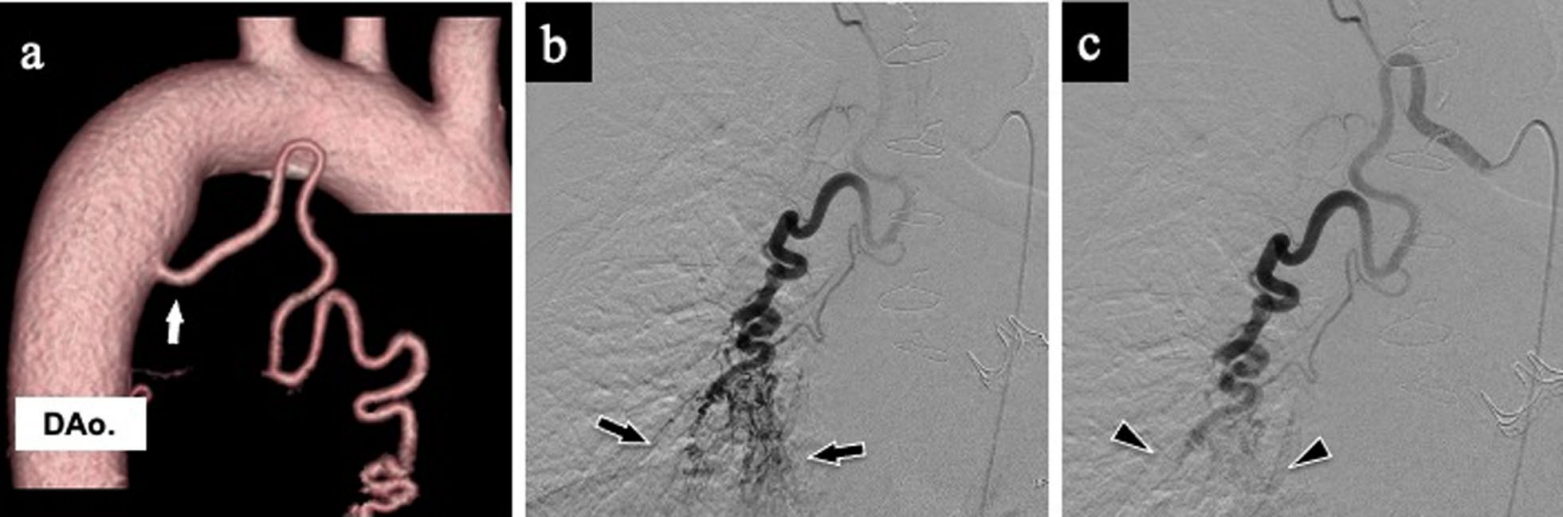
Preoperative computed tomography and angiography images in case 2: a BAH identified on preoperative three-dimensional CT image (white arrow); b pulmonary parenchymal blush in the right lower lobe demonstrated on BA angiography (arrows); c disappearance of parenchymal blush after embolization using a liquid embolic agent (arrowheads). *BAH* bronchial artery hypertrophy, *CT* computed tomography, *BA* bronchial artery, *DAo* descending aorta
